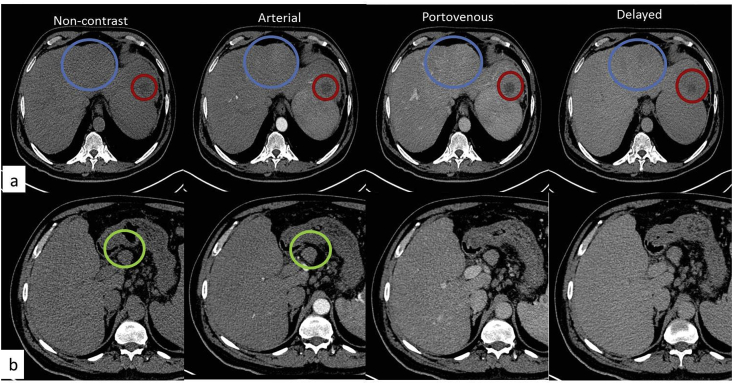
Multiphasic contrast-enhanced CT of liver masses and lymph nodes: (a) axial images at the level of liver showing segment IV two arterially enhancing lesions with delayed washout (typical criteria of HCC) (blue circles), another segment II hypodense non-enhancing lesion through all phases (red circles). (b) Axial images at the level of prominent porta hepatis lymph node (yellow circle) with the absence of hyperenhancement are characteristic features of the primary CD of lymph nodes. (For interpretation of the references to colour in this figure legend, the reader is referred to the Web version of this article.)
